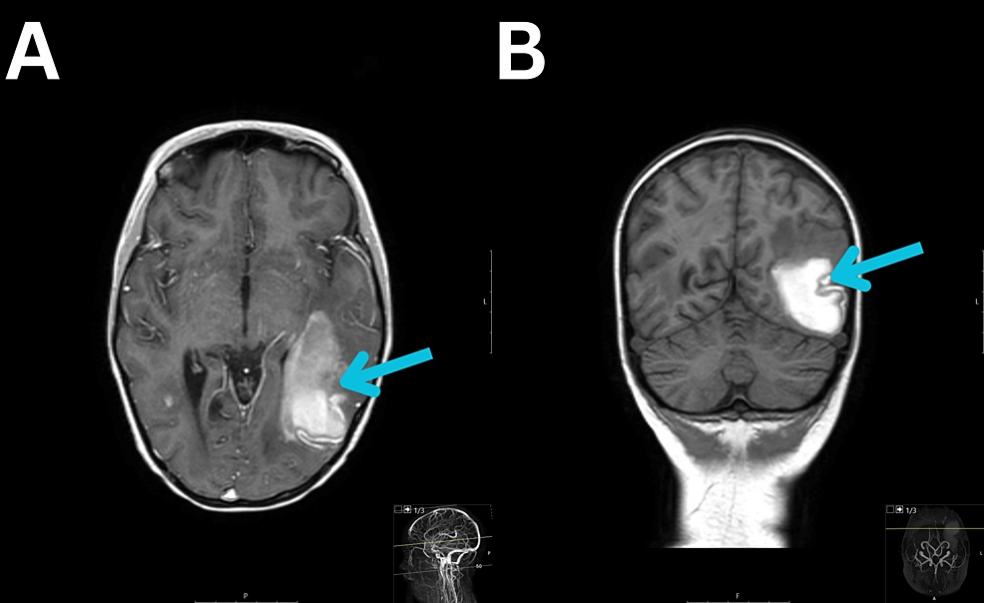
T1-Weighted MRI With and Without Contrast Intracerebral hemorrhage in the left occipitotemporal region (images A and B, blue arrows). MRI, magnetic resonance imaging.
